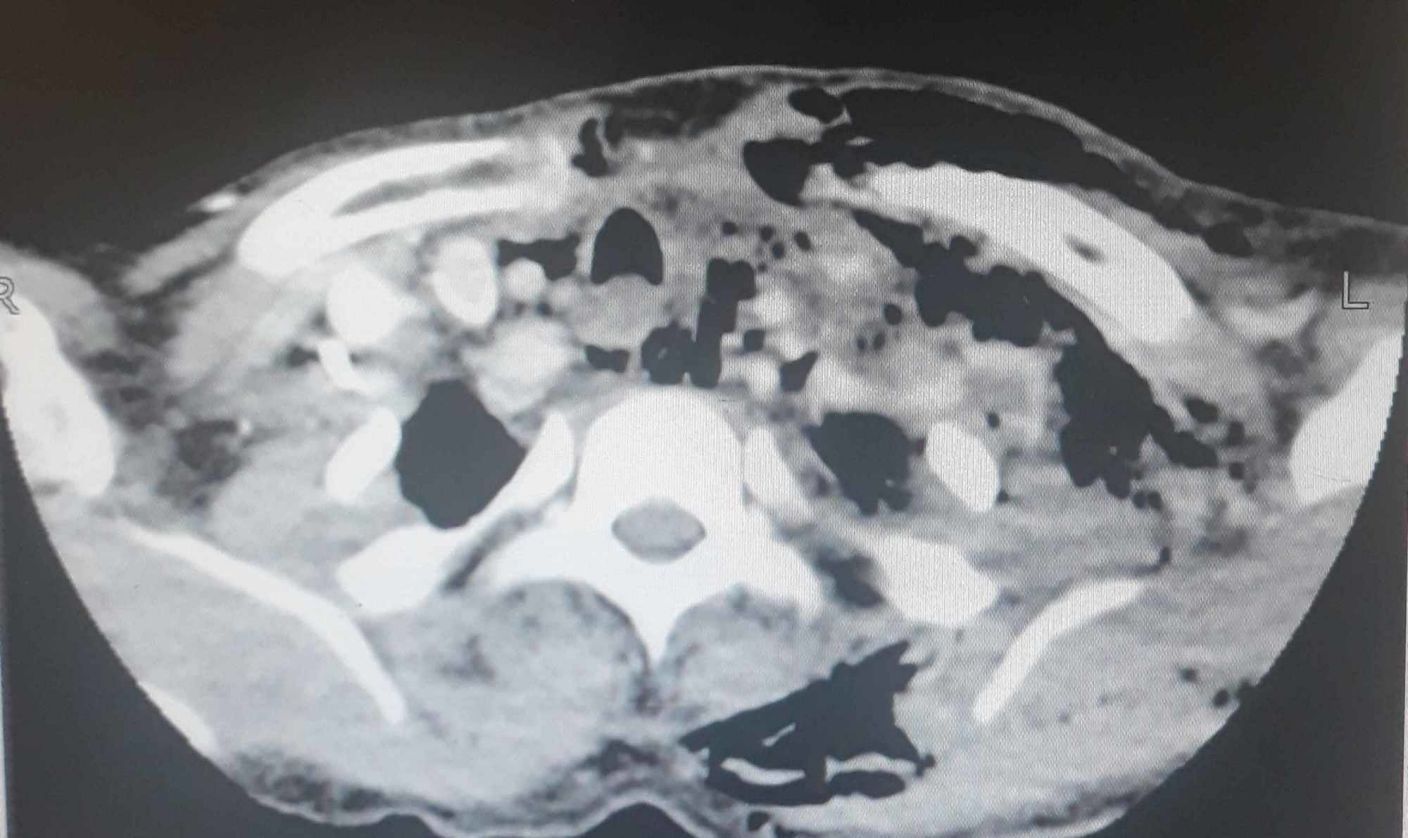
Contrasted computed tomography of the neck showing several multiloculated air pockets in the left paratracheal and prevertebral regions with a focal filling defect in the left internal jugular vein
